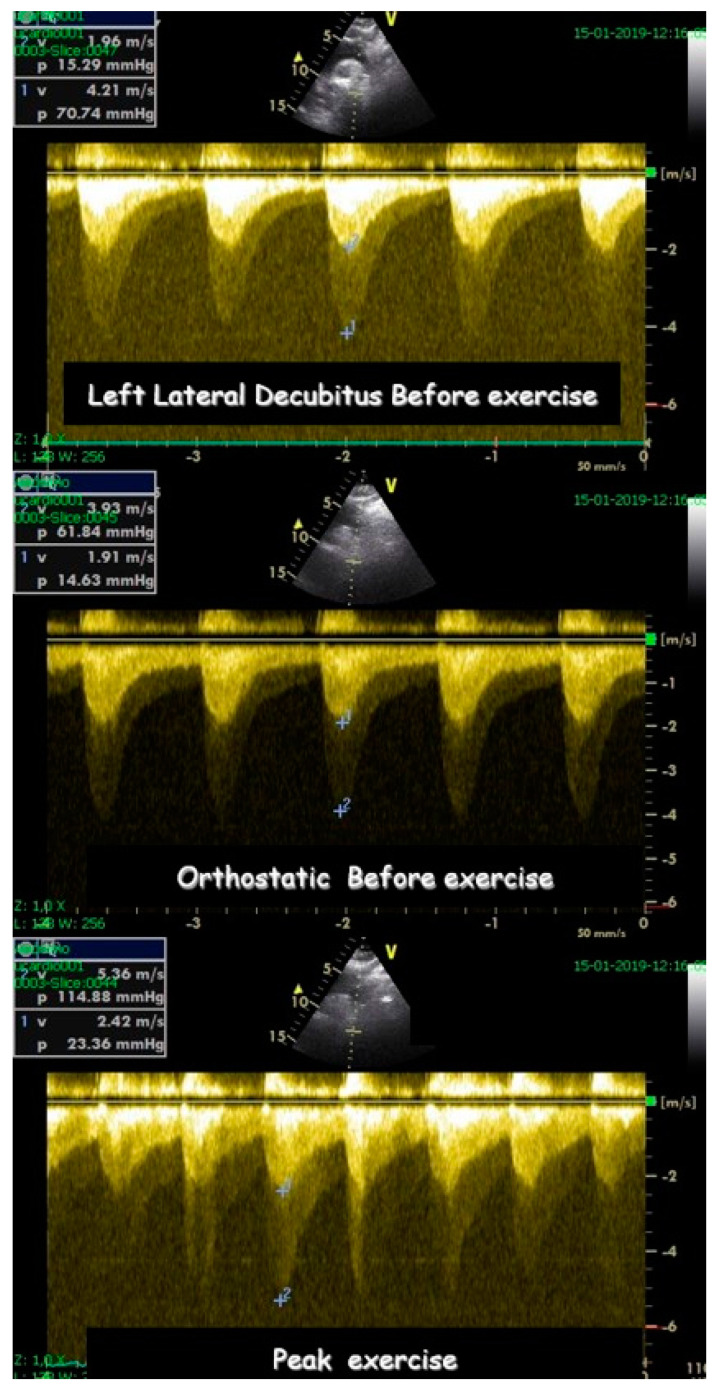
Aortic gradient evaluated in a patient with aortic coarctation previously treated with a stent. Based on the exercise stress echocardiography results, the child was treated again [1].
